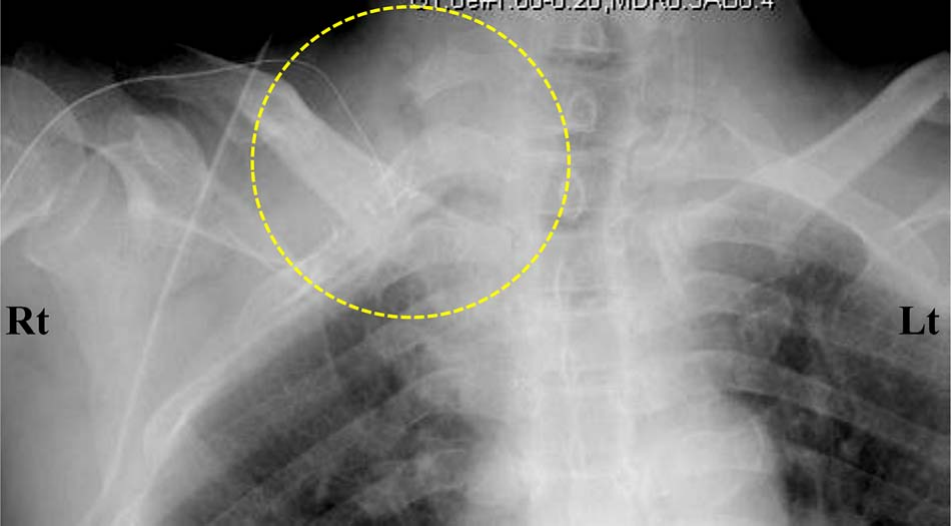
Postoperative cervicothoracic spine X-ray. Anterior–posterior view. The right first and second ribs were surgically resected (circle).
